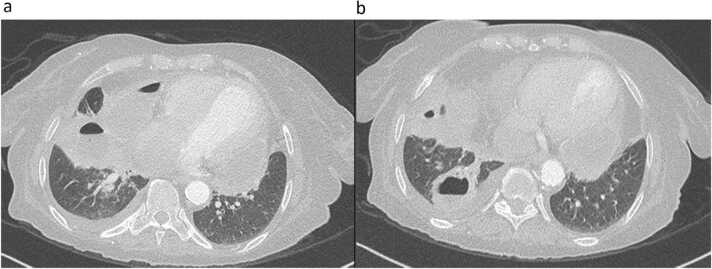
Computed tomography scan of the chest showing multiple cavitary lesions in the right middle and lower lobes of the lung, the largest of which measuring 5.5 × 4.2 × 3.5 cm (a) and a similar right pericardial lesion along the right heart border measuring around 8.5 × 6.2 × 3.6 cm (b).
